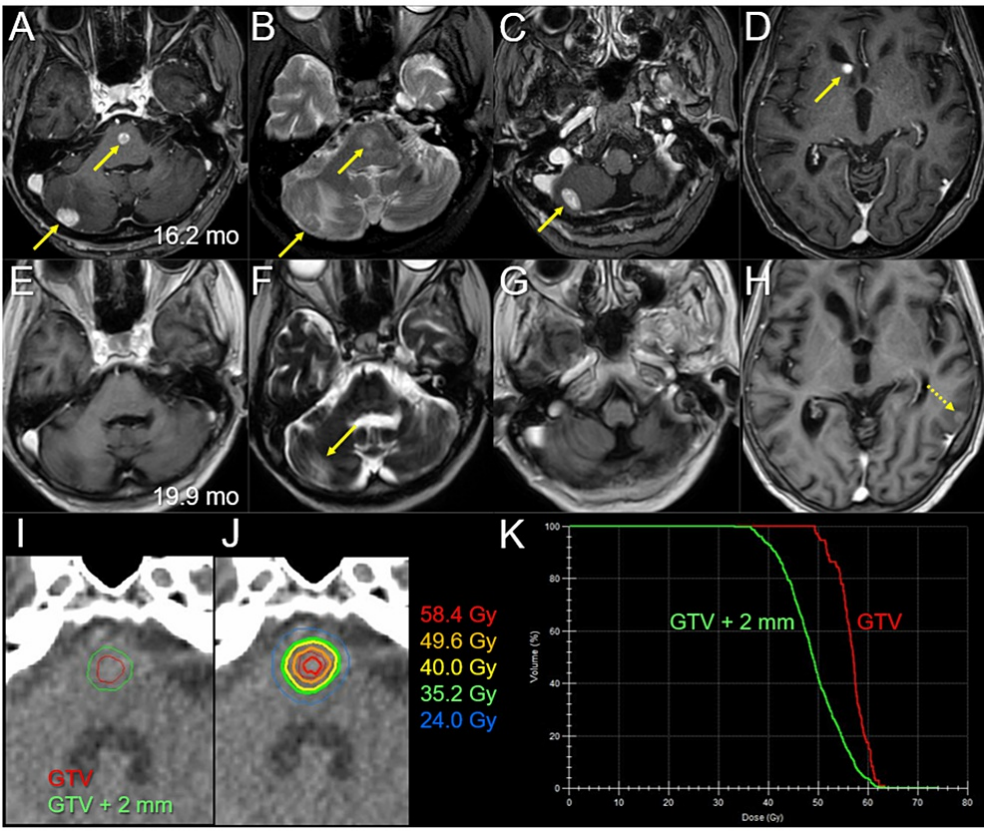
Magnetic resonance images before and after salvage stereotactic radiosurgery The images show axial CE-T1-WIs (A, C-E, G, H); axial T2-WIs (B, F); at 16.2 months (mo) after the initiation of WBRT (A-D); at 19.9 months (3.5 months after the initiation of SRS) (E-H); the target definition of the pons lesion (I); the dose distribution (J); the DVHs (K); and the pons lesion (I-K). (A–H) These images are shown at the same magnification and coordinates under co-registration and fusions. (A-D) Four solid-enhancing lesions (arrows in A, C, and D) are associated with perilesional edema (arrows in B). (E-H) The four lesions regressed completely, only leaving faint perilesional edema (arrow in F); however, a new lesion developed (dashed arrow in H). (J) Five representative isodose lines and the GTV contour are superimposed onto an axial CT image. CE: contrast-enhanced; WIs: weighted images; mo: months; WBRT: whole-brain radiotherapy; DVHs: dose-volume histograms; GTV: gross tumor volume; GTV + 2 mm: the reference volume generated by adding an isotropic 2-mm margin to a GTV
